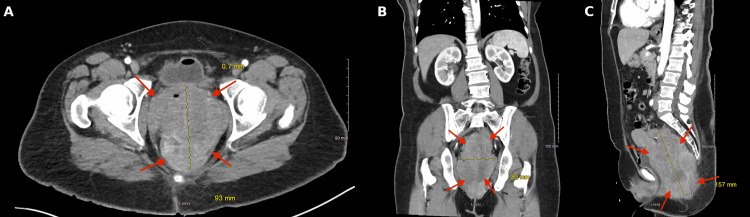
Contrast-enhanced CT of the abdomen and pelvis in the (A) axial, (B) coronal, and (C) sagittal views Contrast-enhanced CT demonstrates a large soft-tissue mass within the posterior pelvis. Arrows indicate the pelvic mass measuring approximately 93×83×157 mm occupying the rectouterine space. The mass displaces the urinary bladder and exerts a substantial pelvic mass effect, contributing to compression of adjacent vascular and urinary structures. CT, computed tomography
